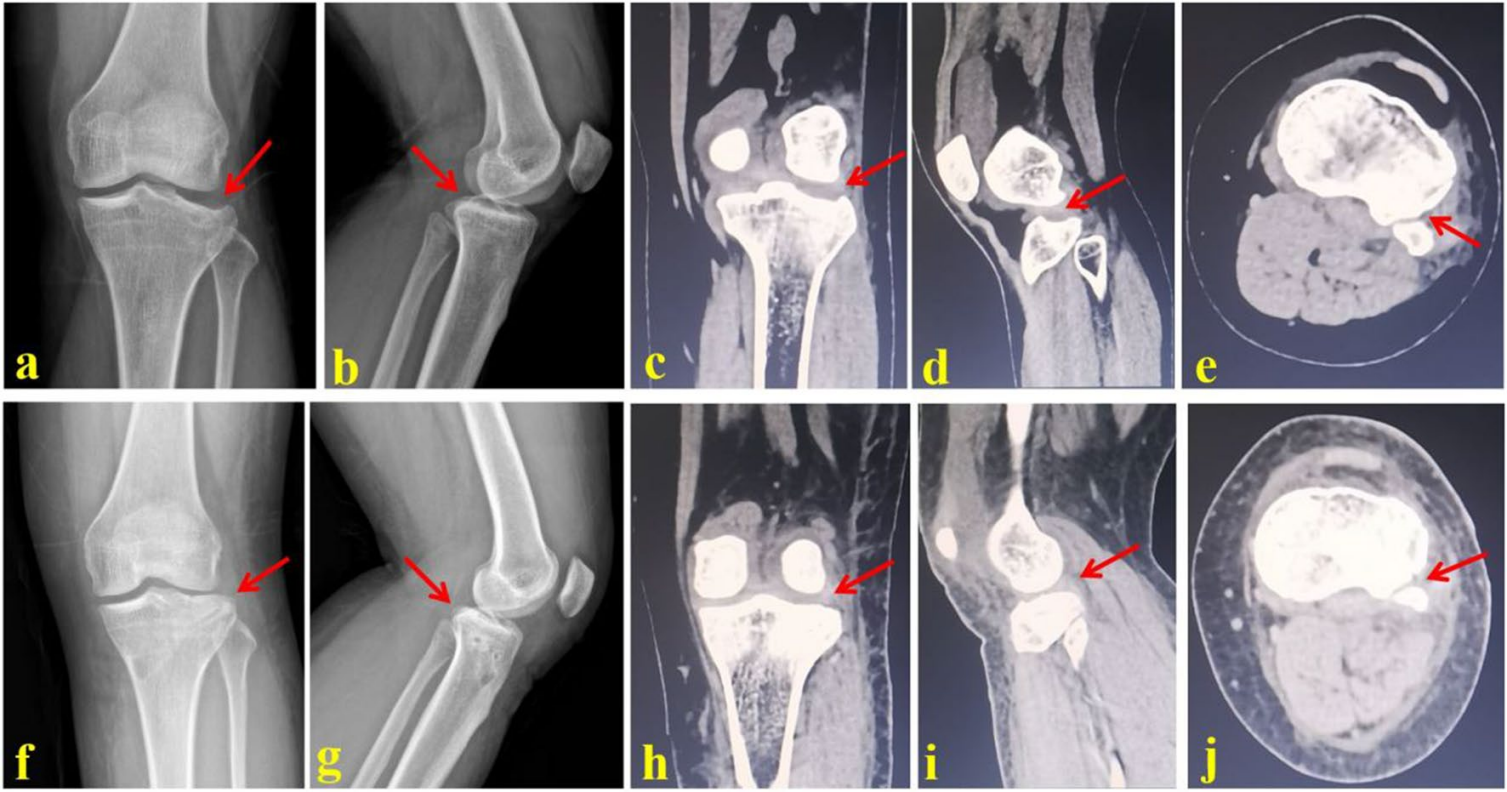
A 35-year-old female suffered from the PL-depression tibial plateau fracture and was successfully treated by the PSO technique. (a,b): AP and lateral X-ray images of the injured tibial plateau; (c–e) Coronal, horizontal and sagittal CT scan images before operation; (f,g) AP and lateral X-ray images of the tibial plateau after operation; (h–j) Coronal, horizontal and sagittal CT scan images after operation. Red arrows indicate the PL-depression fragment of the tibial plateau.
